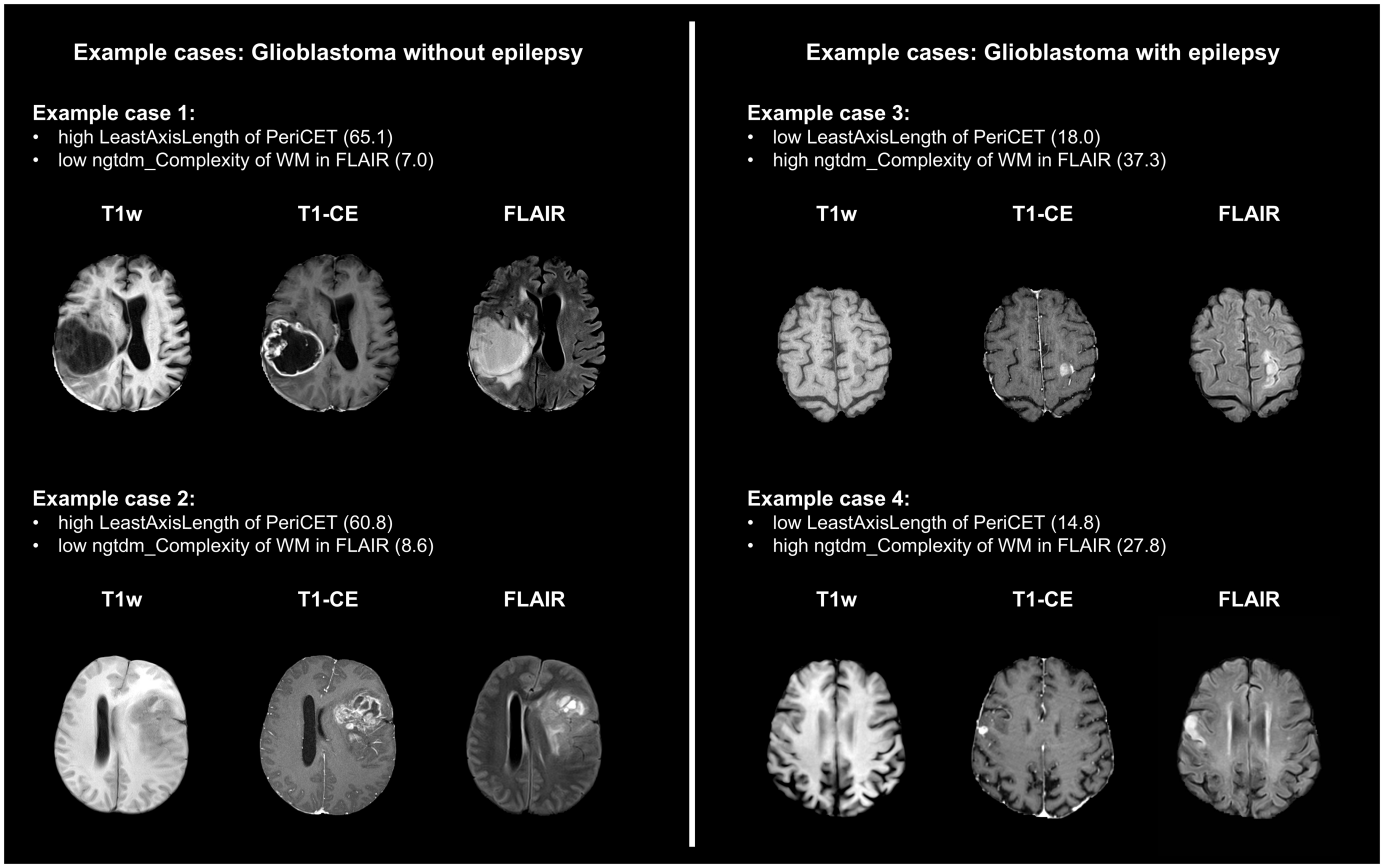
Four example cases are presented here with prominent values for radiomics features, which were important for the classification of epilepsy-associated glioblastoma. LeastAxisLength was the most important feature for models trained with only features from the peritumor region (PeriCET). For the white matter region (WM), complexity from neighborhood gray-tone difference matrix (ngtdm) in FLAIR was the most relevant feature.
